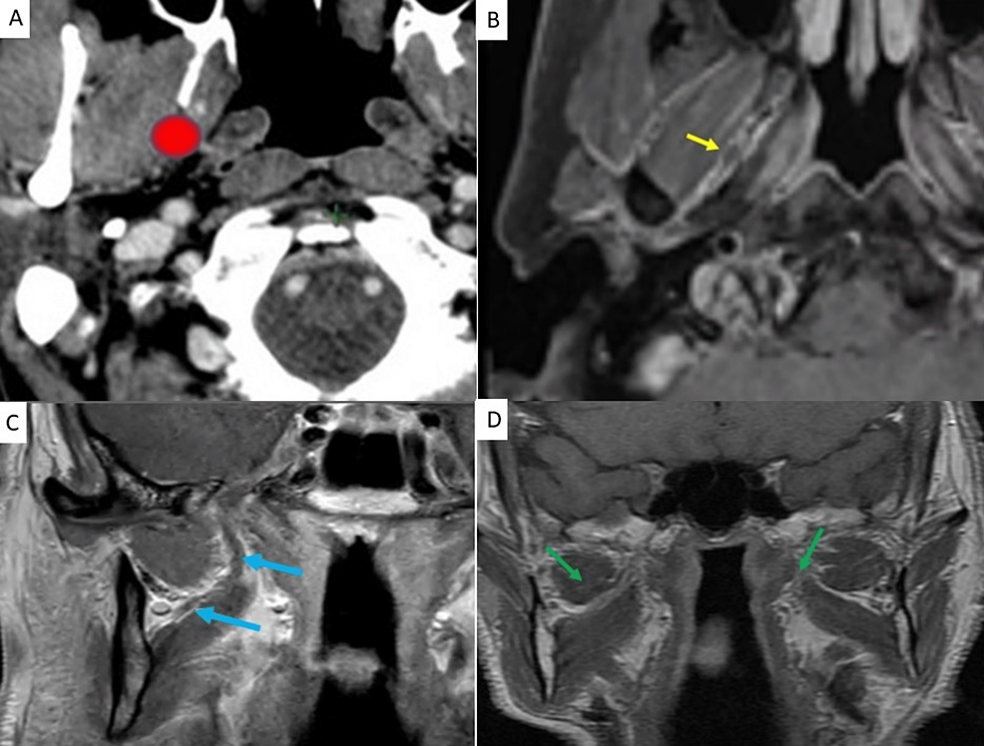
Anchor point at the level of lateral pterygoid A: Location of V3 (red) on axial CT shows how V3 travels medial to the lateral pterygoid muscle and lateral to the tensor veli palatini muscle B: Location of V3 (yellow) on axial fat-suppressed contrast-enhanced T1W MRI C: Location of V3 medial to the lateral pterygoid muscle (blue arrows) on coronal fat-suppressed contrast-enhanced T1 MRI D: Retrograde recurrence along a lingual nerve on contrast-enhanced coronal T1 MRI. The green arrow on the right shows the normal appearance of the left lingual nerve coursing between the medial and lateral pterygoid muscles. Green arrow on the left shows diffuse enhancement and thickening of the right lingual nerve due to perineural tumor spread
